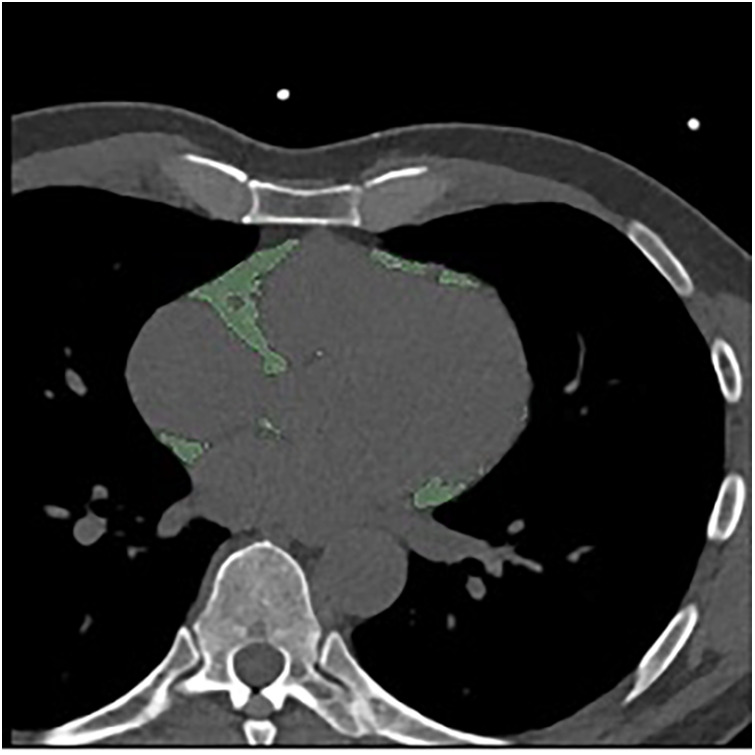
Example CT segmentation of a 69-year-old male patient with epicardial adipose tissue highlighted in green.
